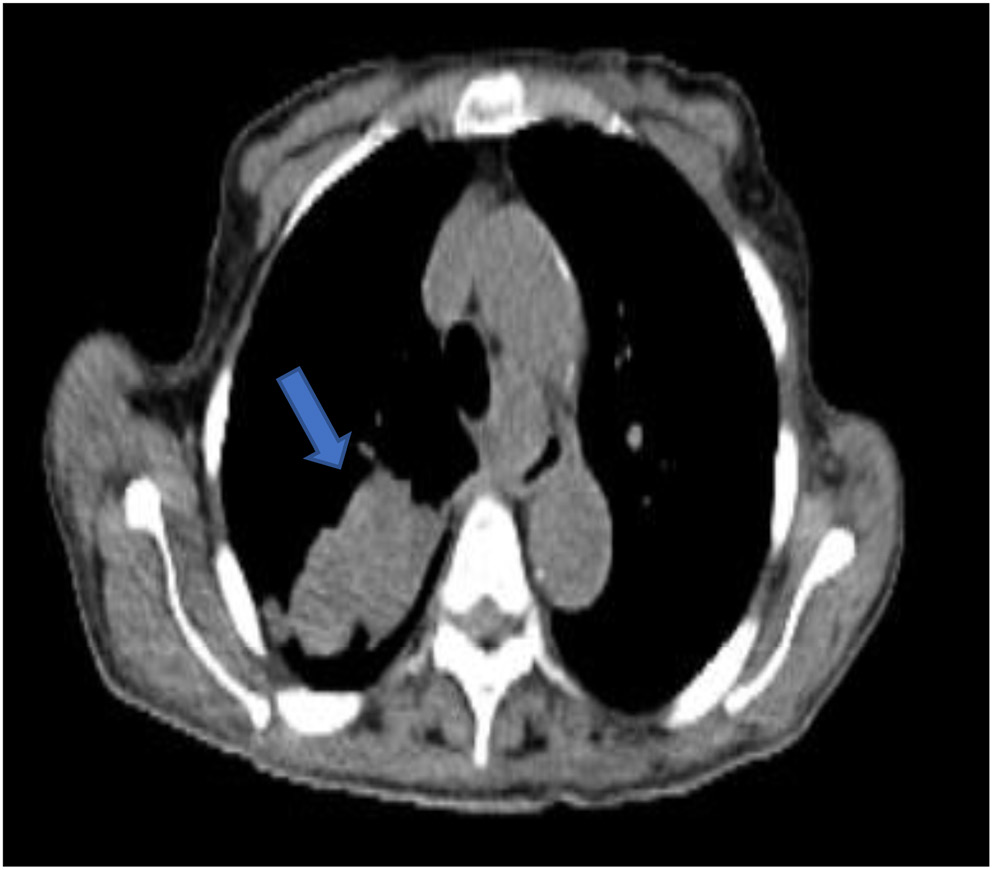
CT scan with transverse plane view of the 4 × 4.5 cm right upper lobe lung lesion.
